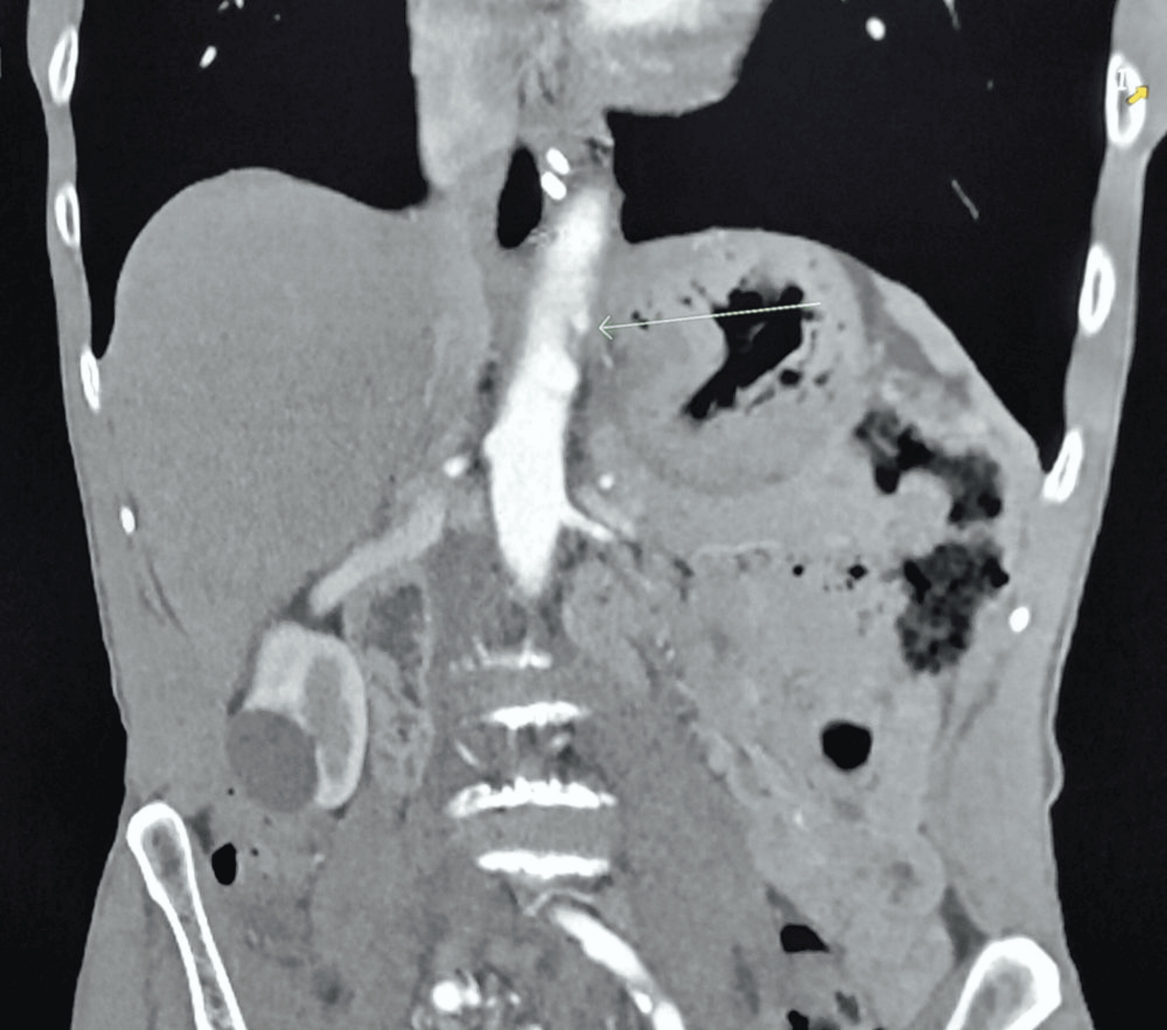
CT angiography of the chest, abdomen, and pelvis, with the green arrow showing severe proximal celiac trunk stenosis.
